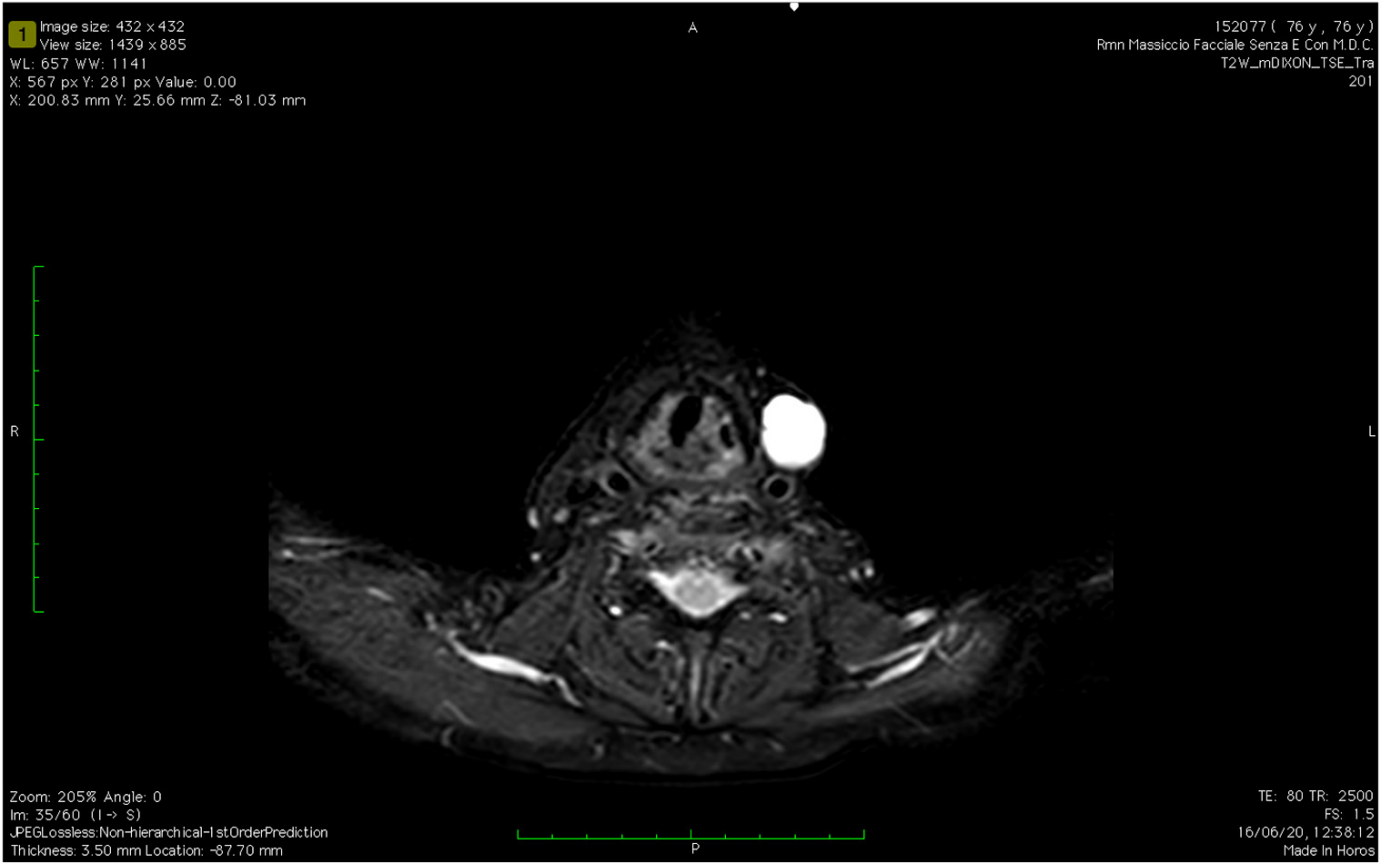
Sixteen months MRI follow up (axial view). Non‐enhancing ovalar mass (2 × 2 cm) in the left thyrohyoid membrane.
